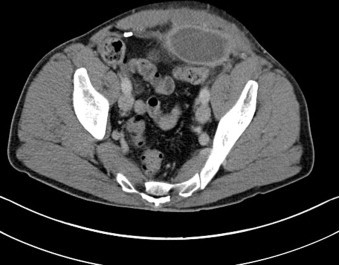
Computed tomography image showing a left sided collection at the site of a laparoscopically inserted hernia repair mesh.
